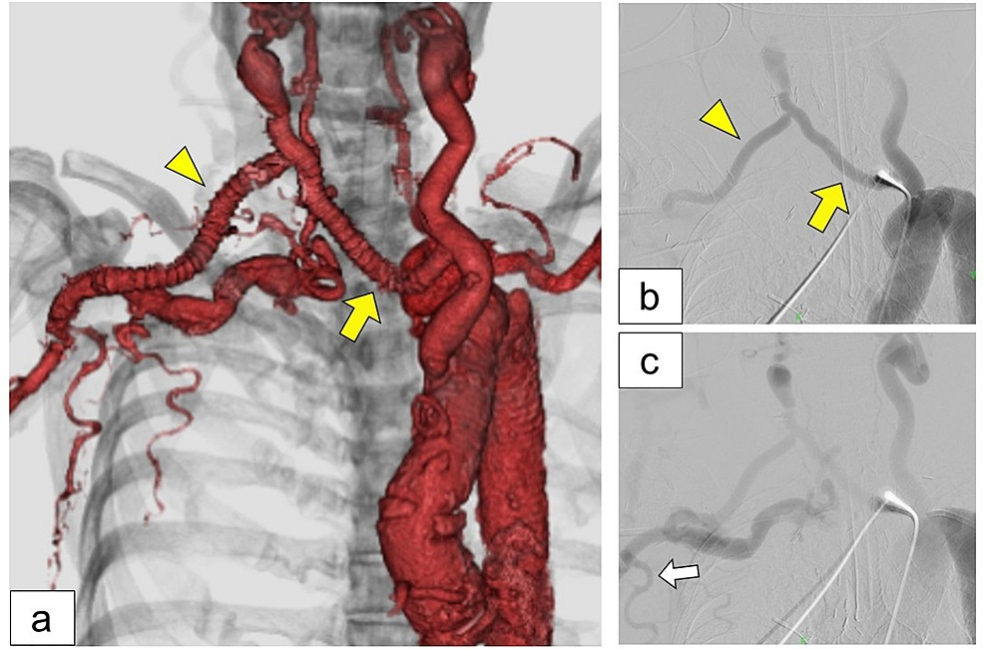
After the extra-anatomical bypass operation. (a) Postoperative computed tomography angiography demonstrated a new prosthetic graft that connected the left common carotid artery and right subclavicular artery (yellow arrowhead) via the right common carotid artery (yellow arrow). (b, c) Postoperative angiography of the ascending aorta revealed anterograde blood flow in the new graft (yellow arrow and arrowhead). The white arrow indicates the right thoracodorsal artery.
